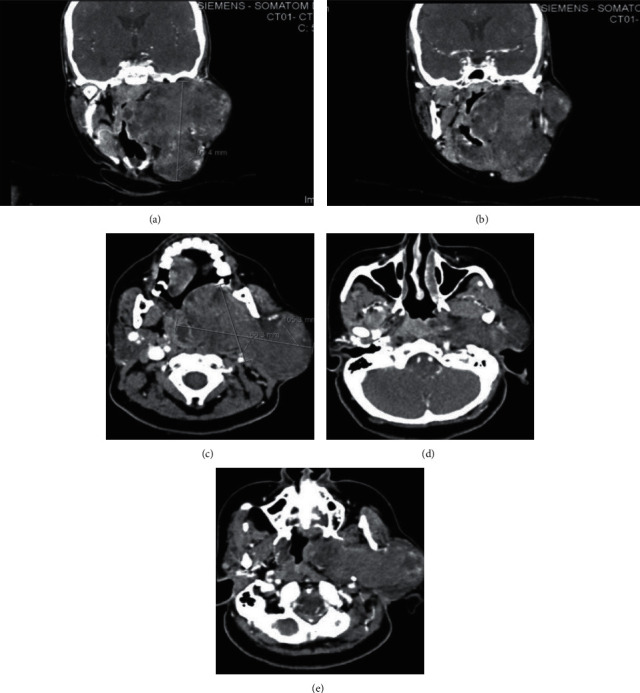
(a, b) Axial cut CT images showing the heterogenous left-sided smooth surface mass with minimum mass effect on the oropharyngeal airway, with no destruction of the mass and no intracranial extension. Mass appears to be around 10 cm in size. (c, d) Coronal cut CT images showing the mass from the left side with no mass effect on oropharyngeal airway.
